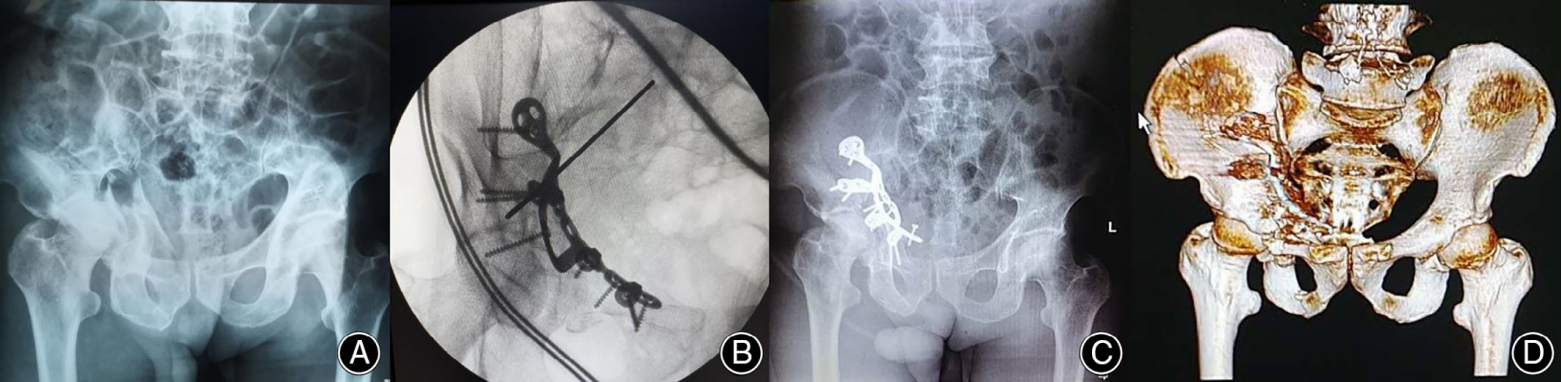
A male with both column fracture. (A) Preoperative radiograph of anteroposterior view; (B) Intraoperative radiograph of obturator oblique view; (C, D) A radiograph of anteroposterior and three‐dimensional reconstruction showed obvious screw loosening during the follow‐up after surgery.
